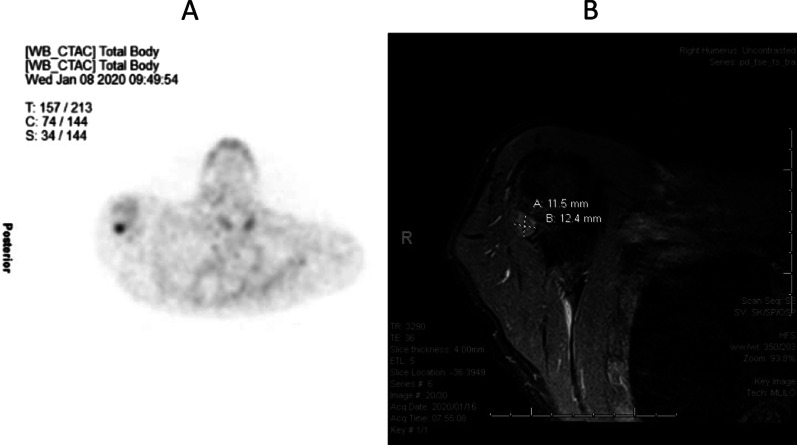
Demonstration of a tumor in the right humeral head by Gallium 68-DOTANOC PET/CT (A) and magnetic resonance imaging (MRI) (B)
